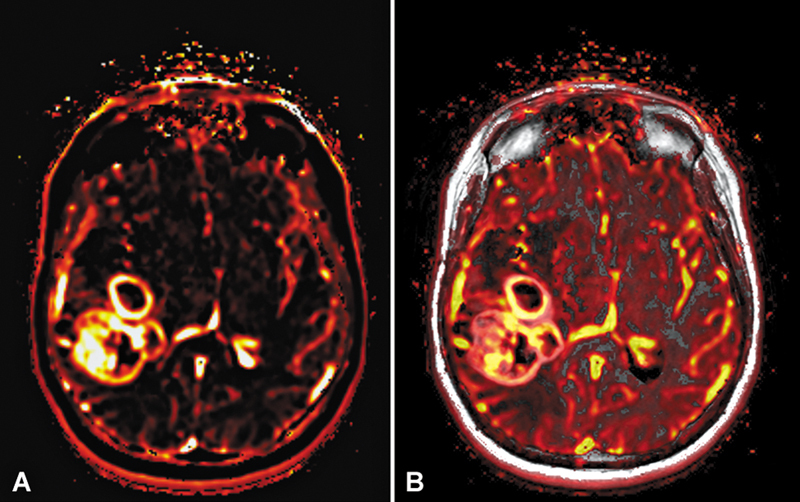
Dynamic contrast-enhanced MRI (DCE-MR), with K
